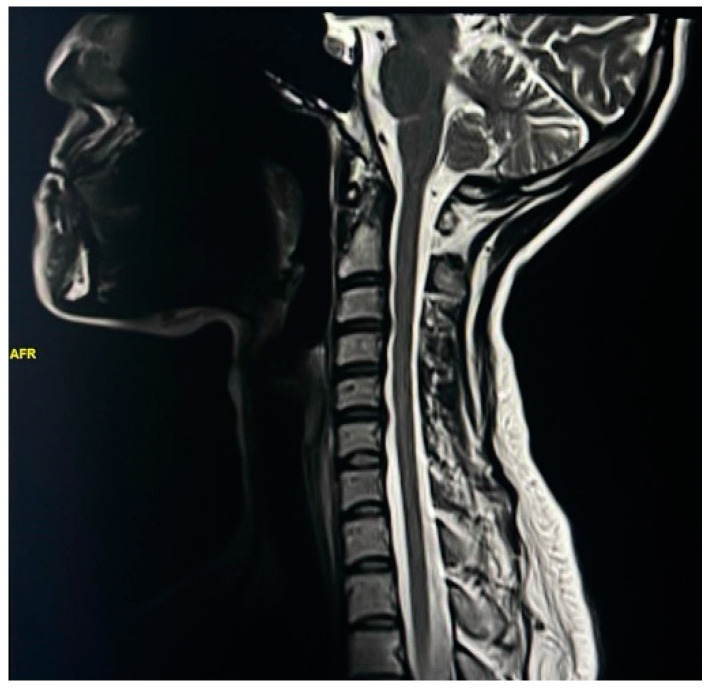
T2W MR image of cervical curve straightening. The image demonstrates straightening of the cervical spine curvature of a 34-year-old male patient with MS.
